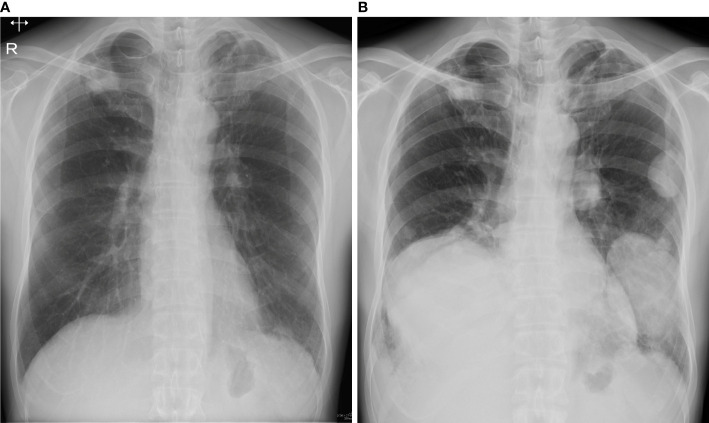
(A) At the time of second surgery, no pulmonary lesion was detected on radiography. (B) Three years after the second surgery, radiography showed multiple lung nodules.
